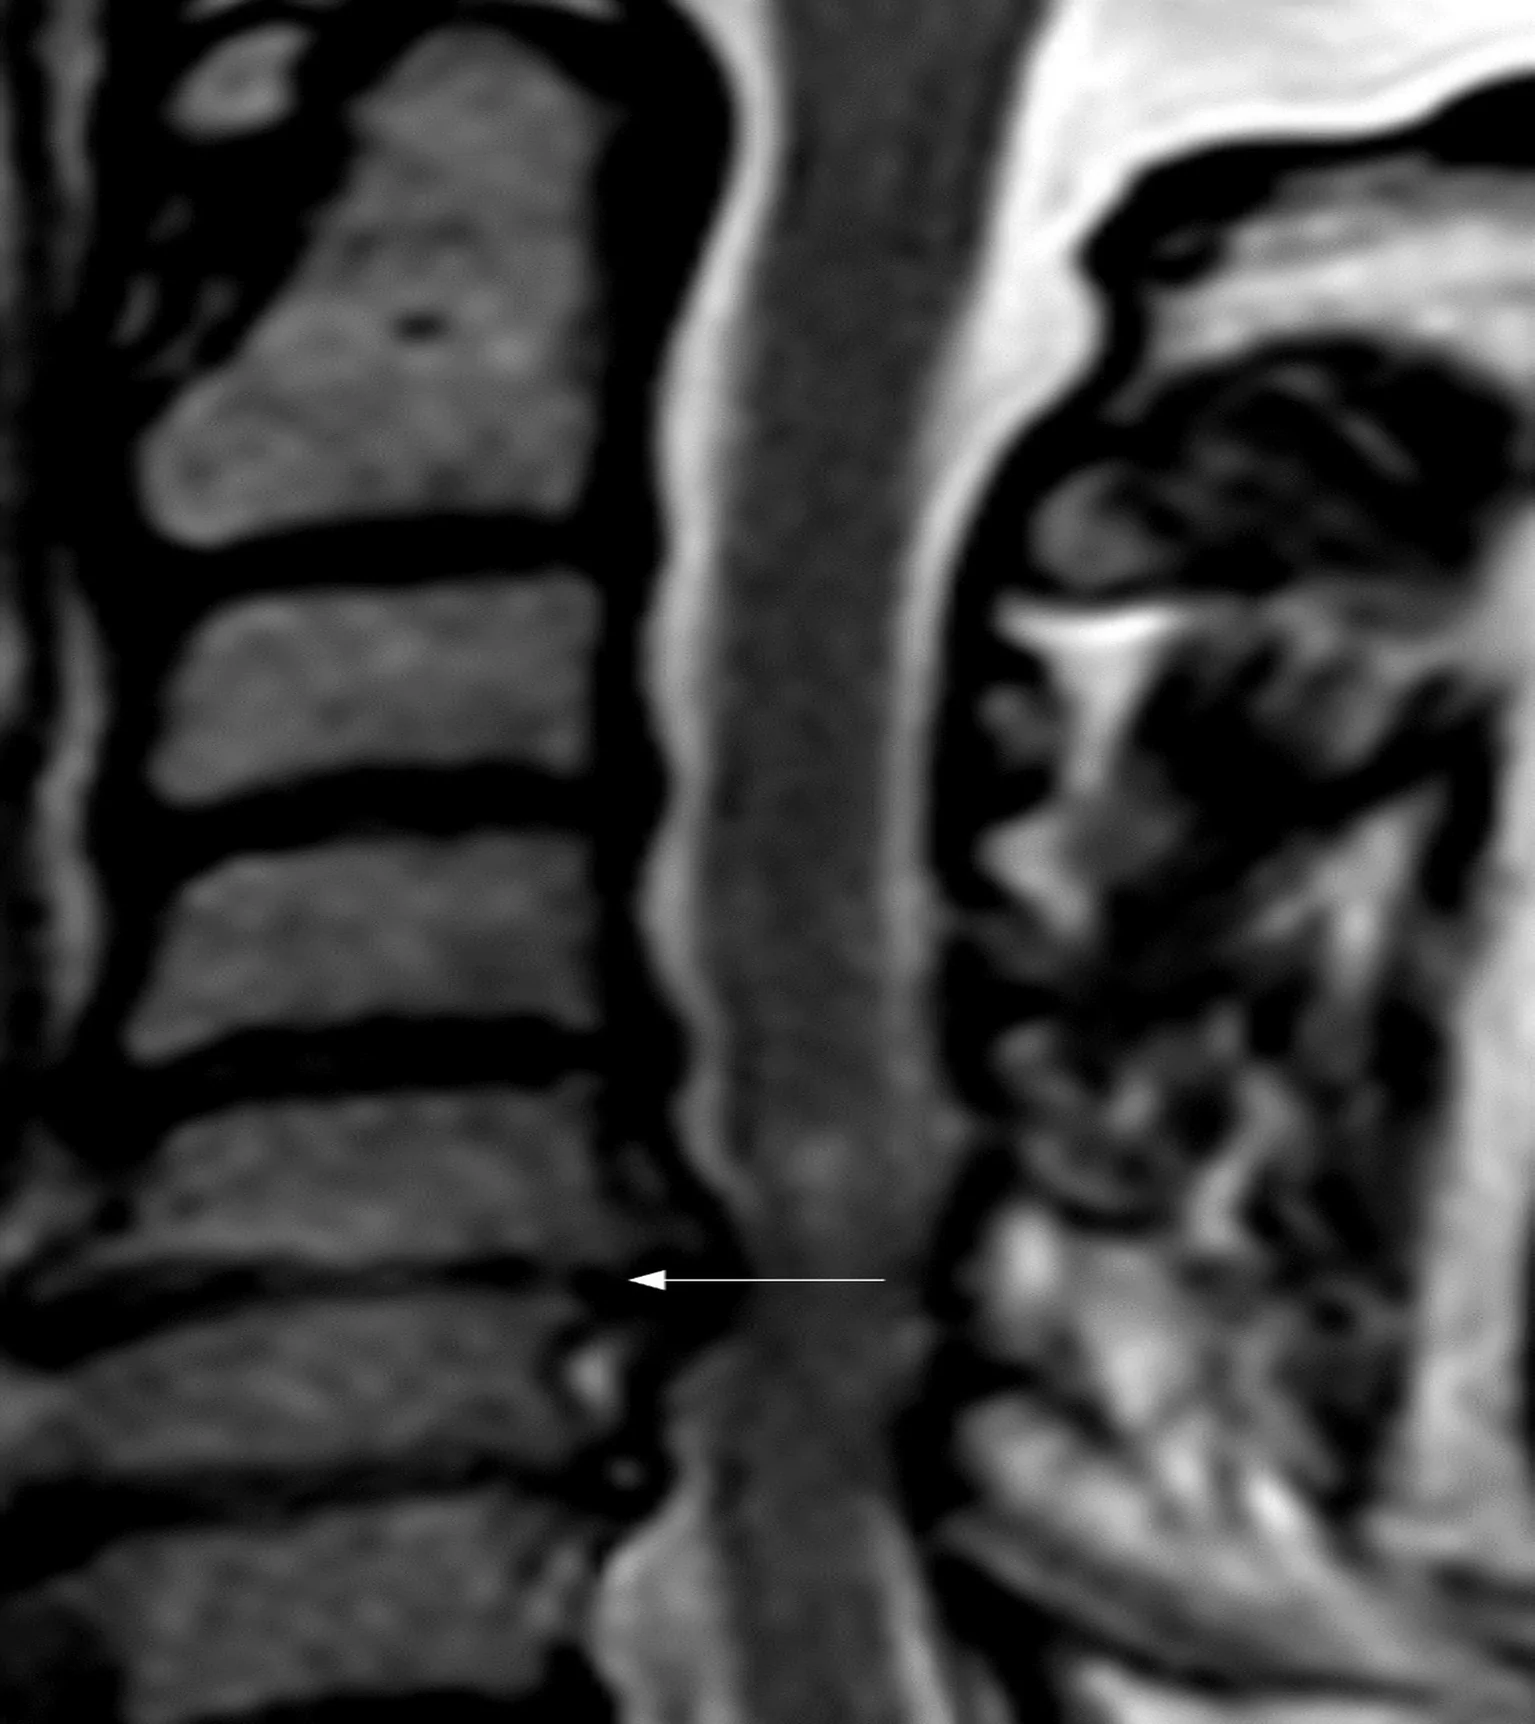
Representative Pfirrmann grade 5 cervical disc degeneration. Mid-sagittal T2-weighted MRI showing severe degeneration at the C5–6 level with associated Modic changes. The operated level exhibits a higher Pfirrmann grade compared with the adjacent discs.
